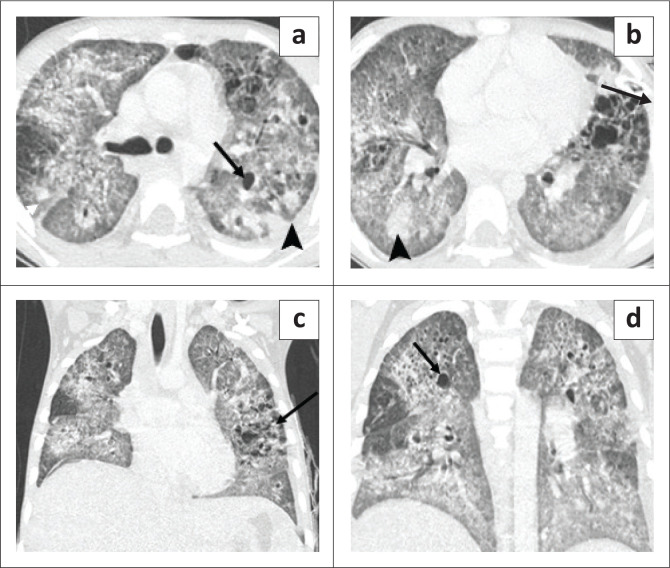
Contrast-enhanced CT chest axial (a, b) and coronal (c, d) images reveal extensive ground glass opacities and confluent nodular opacities in both lungs, along with patchy consolidation in both lower lobes (arrowhead), small lung cysts (arrows), pulmonary interstitial emphysema in the left upper lobe (long arrow in c). Left-sided intercostal drainage is in situ (arrow in b).
